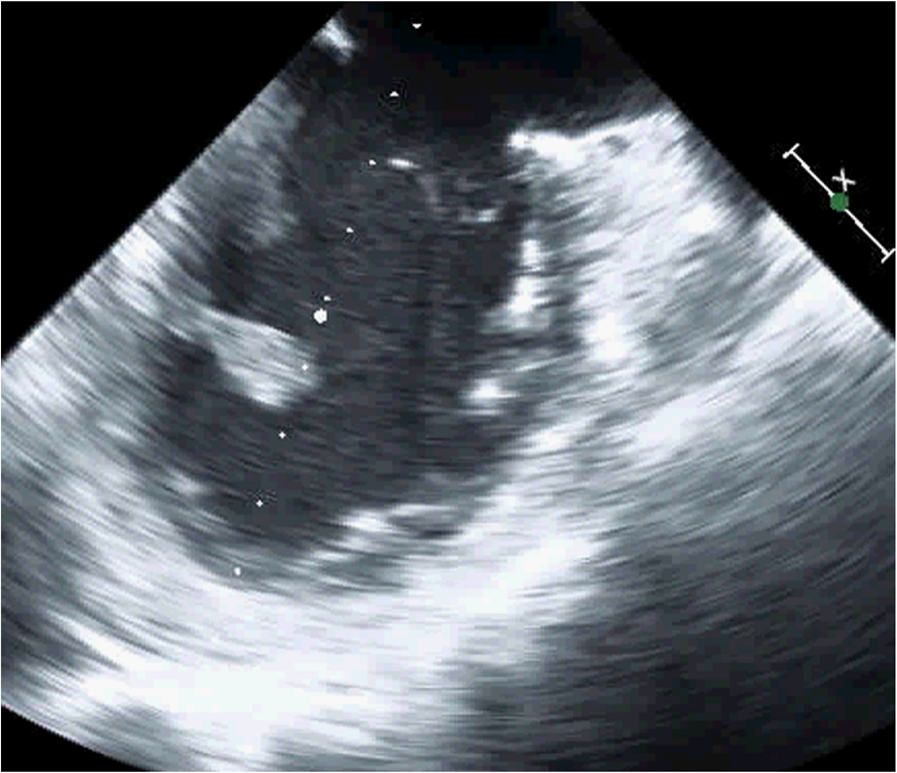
Echocardiogram demonstrating a pedunculated left ventricular vegetation
